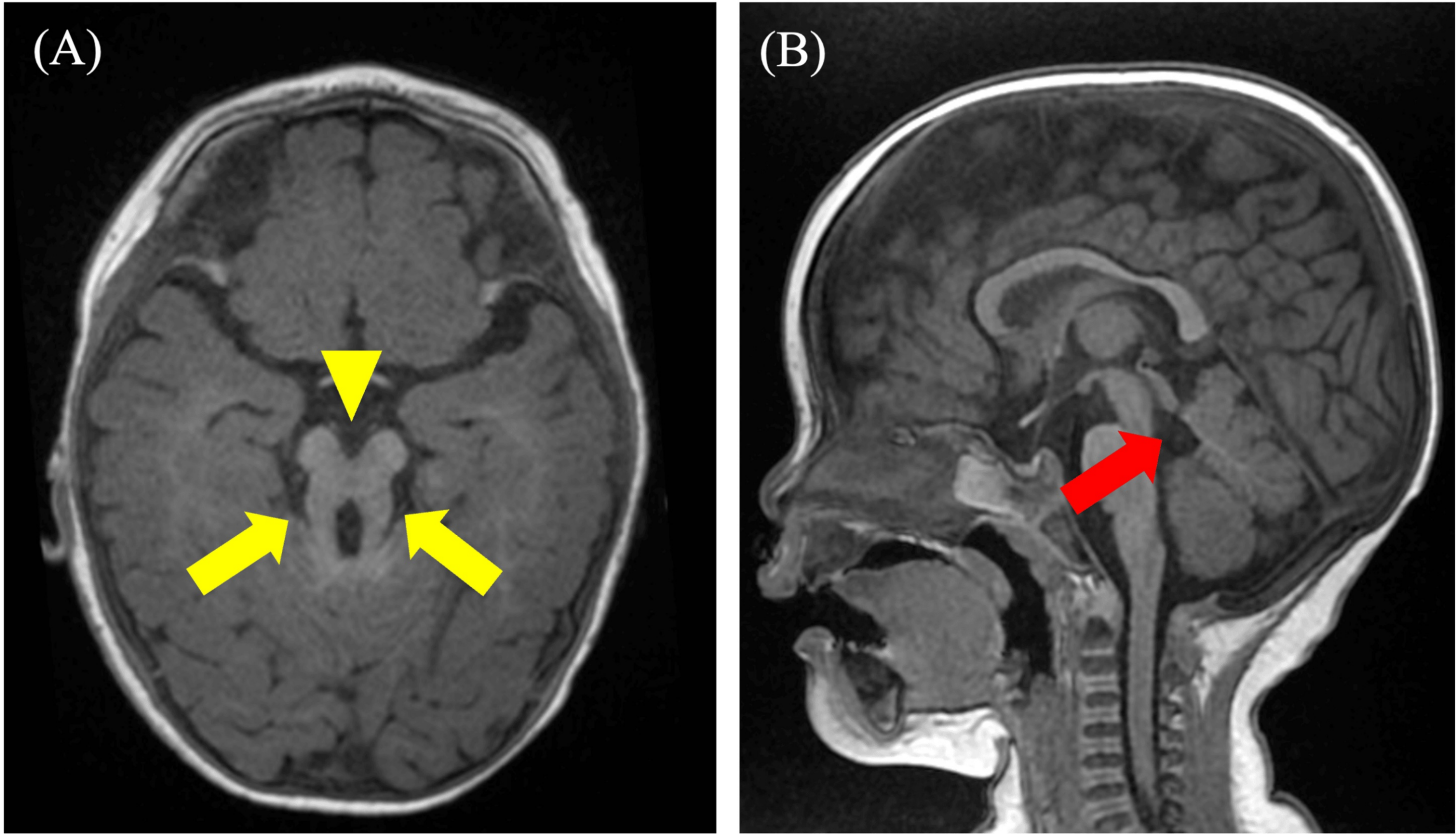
Non-contrast MRI of the head, (A) horizontal section and (B) sagittal section. The molar tooth sign is observed, consisting of a thick, straight superior cerebellar peduncle (yellow arrow), a deep interpeduncular fossa (yellow arrowhead), and hypoplasia of the cerebellar vermis (red arrow).
